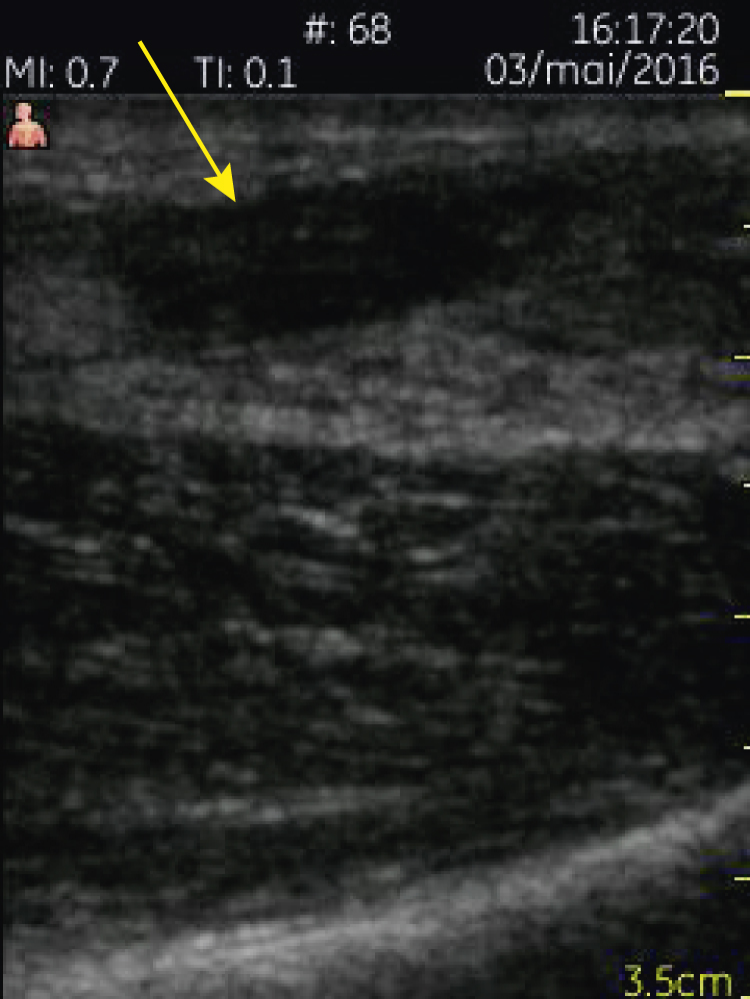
Pocket-sized ultrasound sagittal view of the Achilles rupture.
